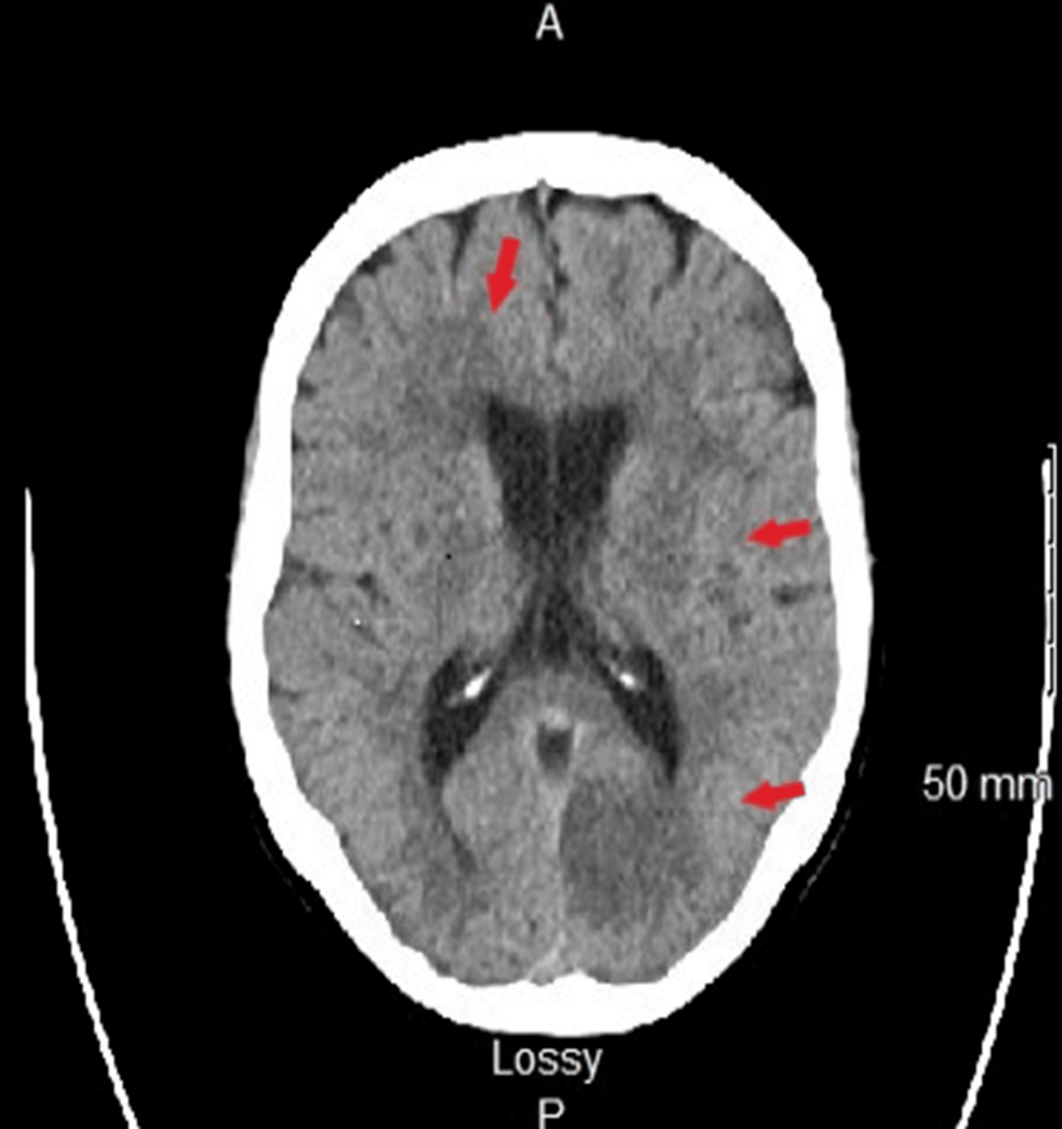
Axial non-contrast CT of the head This image shows patchy hypodensities in the right frontal and left parietal lobes (arrows), consistent with acute infarcts. CT: computed tomography
